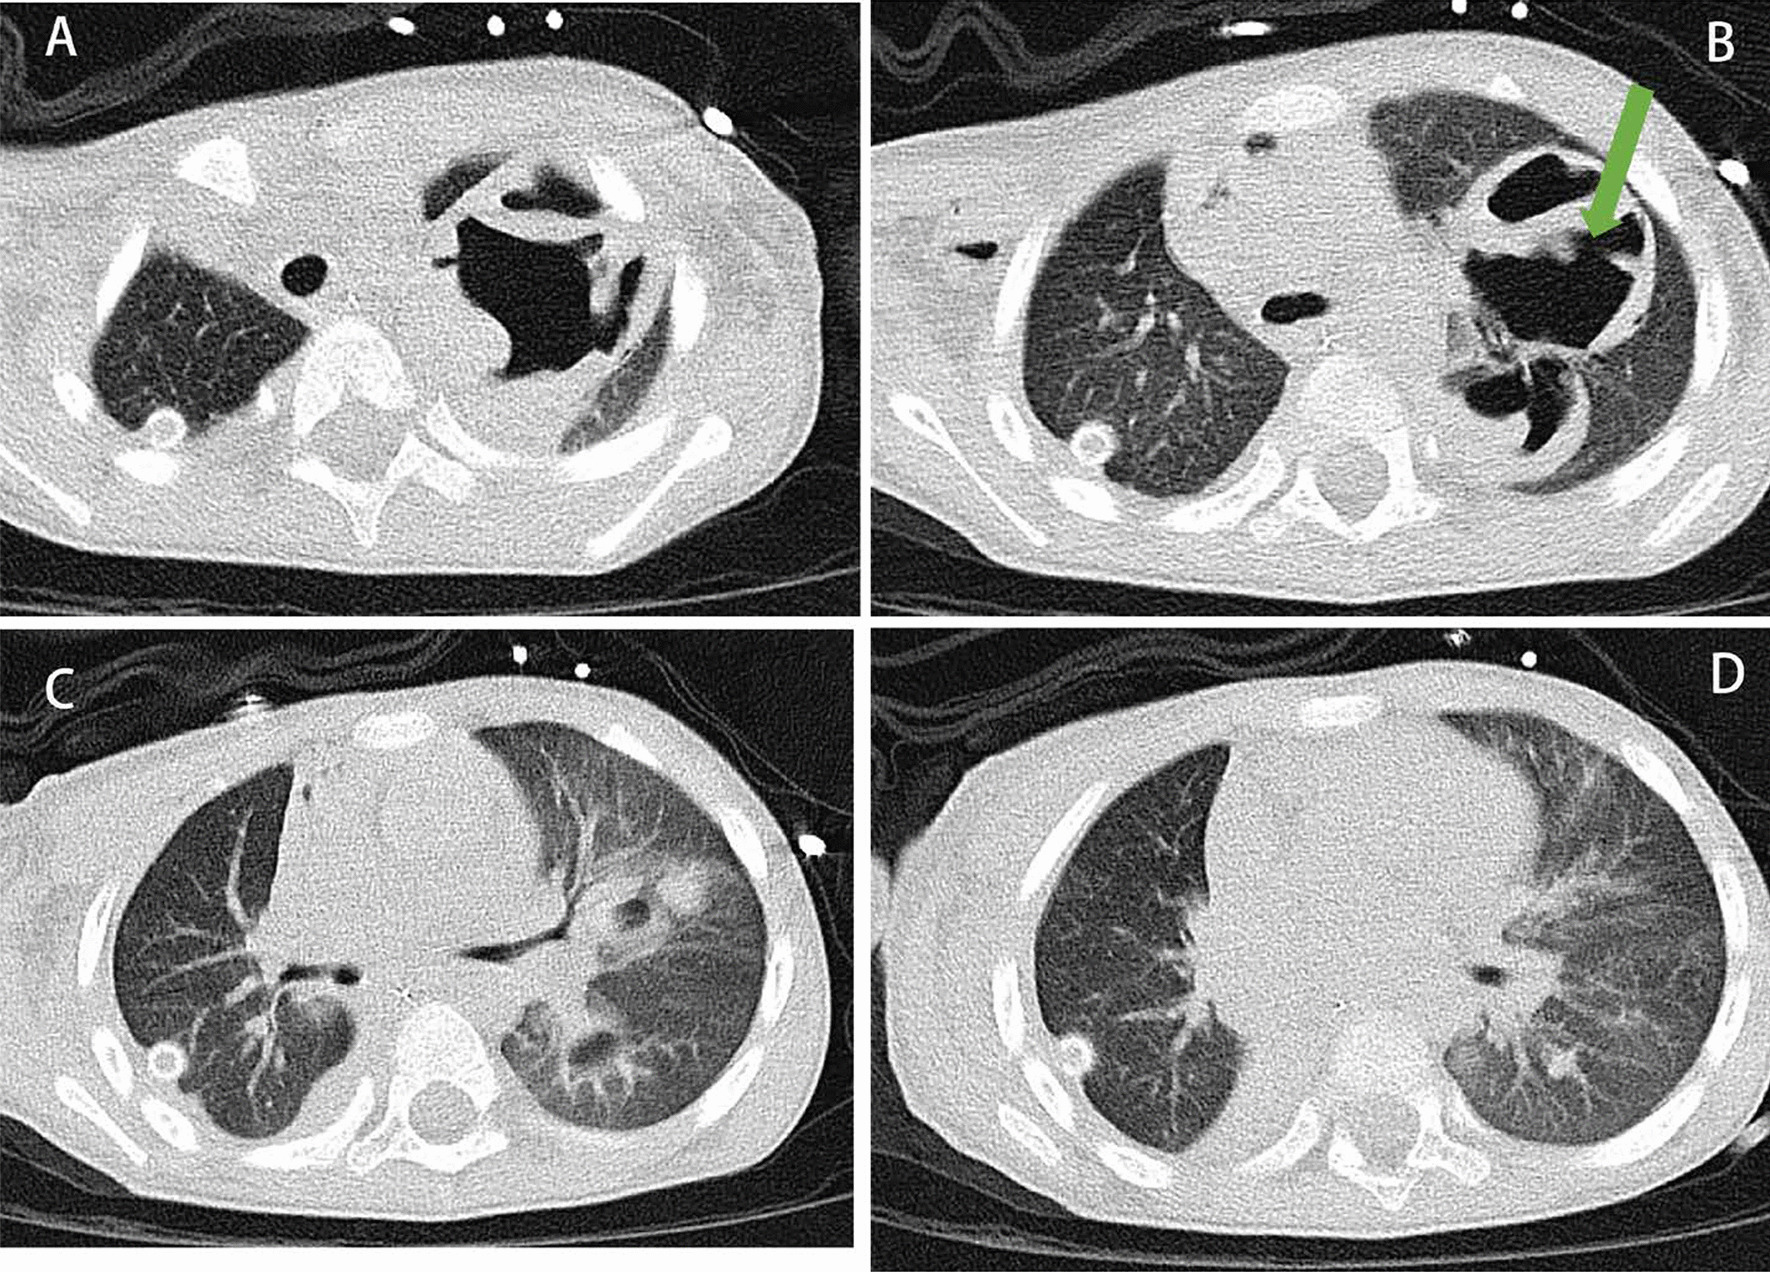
Chest CT (6.24) showed a mass of high-density shadow in the upper lobe of the left lung with cavitation (green arrow)
